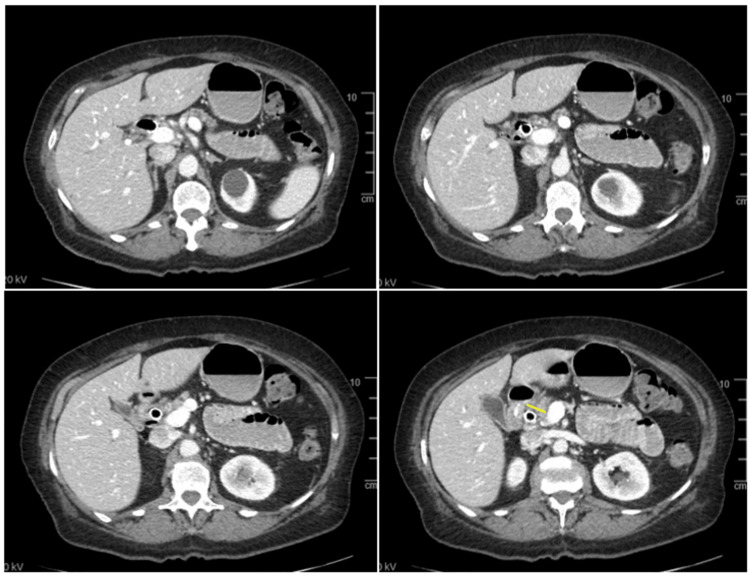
CT imaging of the pancreatic tumour at 6.8 months from diagnosis. The yellow bar indicates the longest tumour diameter CT: computed tomography
